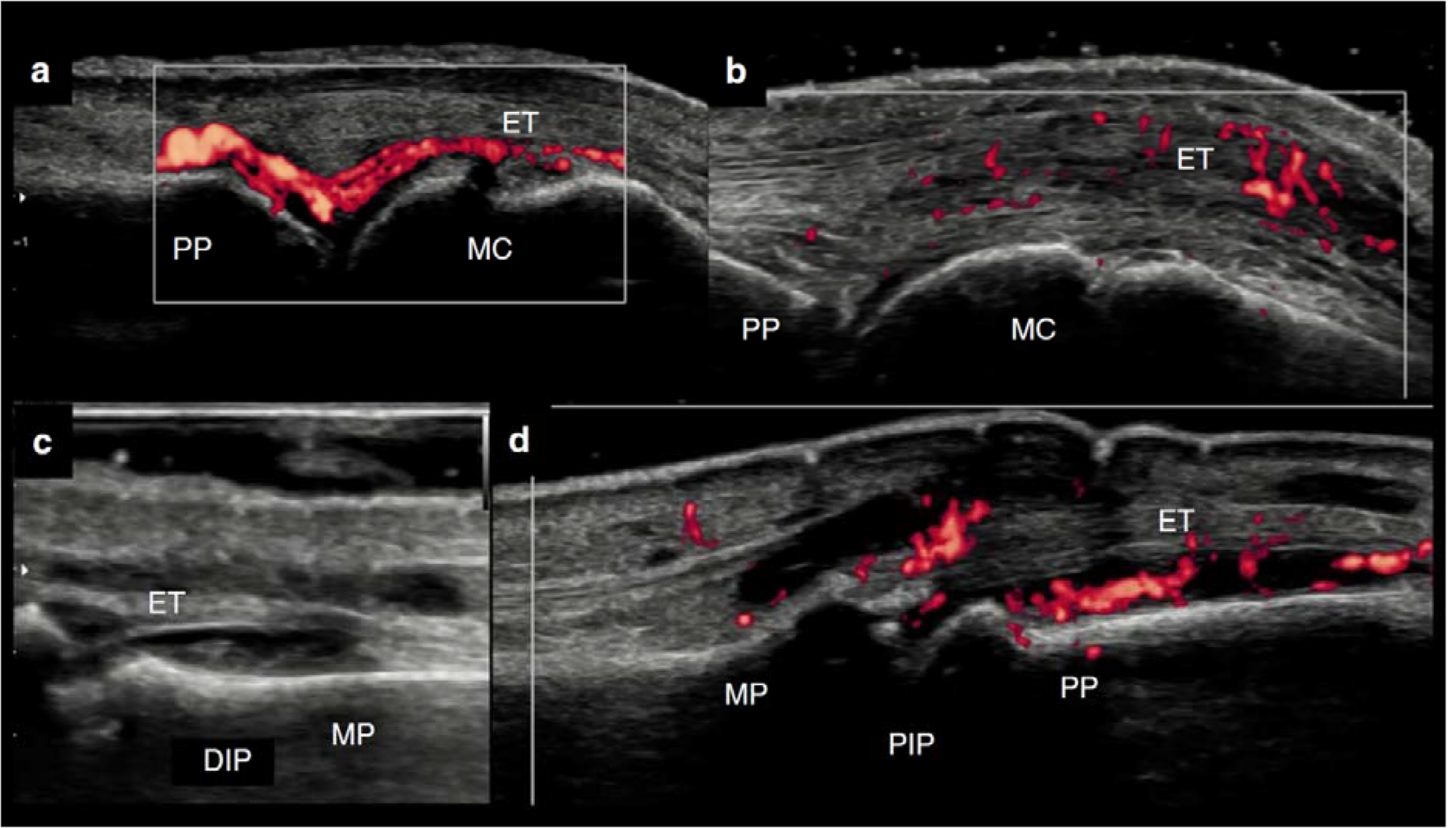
a–b Dorsal scan of the metacarpophalangeal joint by ultrasonography. a Longitudinal view. Active synovitis in an early rheumatoid arthritis (synovium-centric disease). b Longitudinal view. Synovio-entheseal complex (SEC) inflammation (active peritendinitis of the extensor tendon and mild active synovitis) in an early psoriatic arthritis (SEC-centric disease). c Dorsal scan of the distal interphalangeal (DIP) joint by ultrasonography. Longitudinal view. Synovitis of the DIP joint in an early psoriatic arthritis patient. d Dorsal scan of the proximal interphalangeal (PIP) joint by ultrasonography. Longitudinal view. SEC inflammation of the PIP joint (central slip enthesitis of the extensor tendon and active synovitis). *ET* extensor tendon
